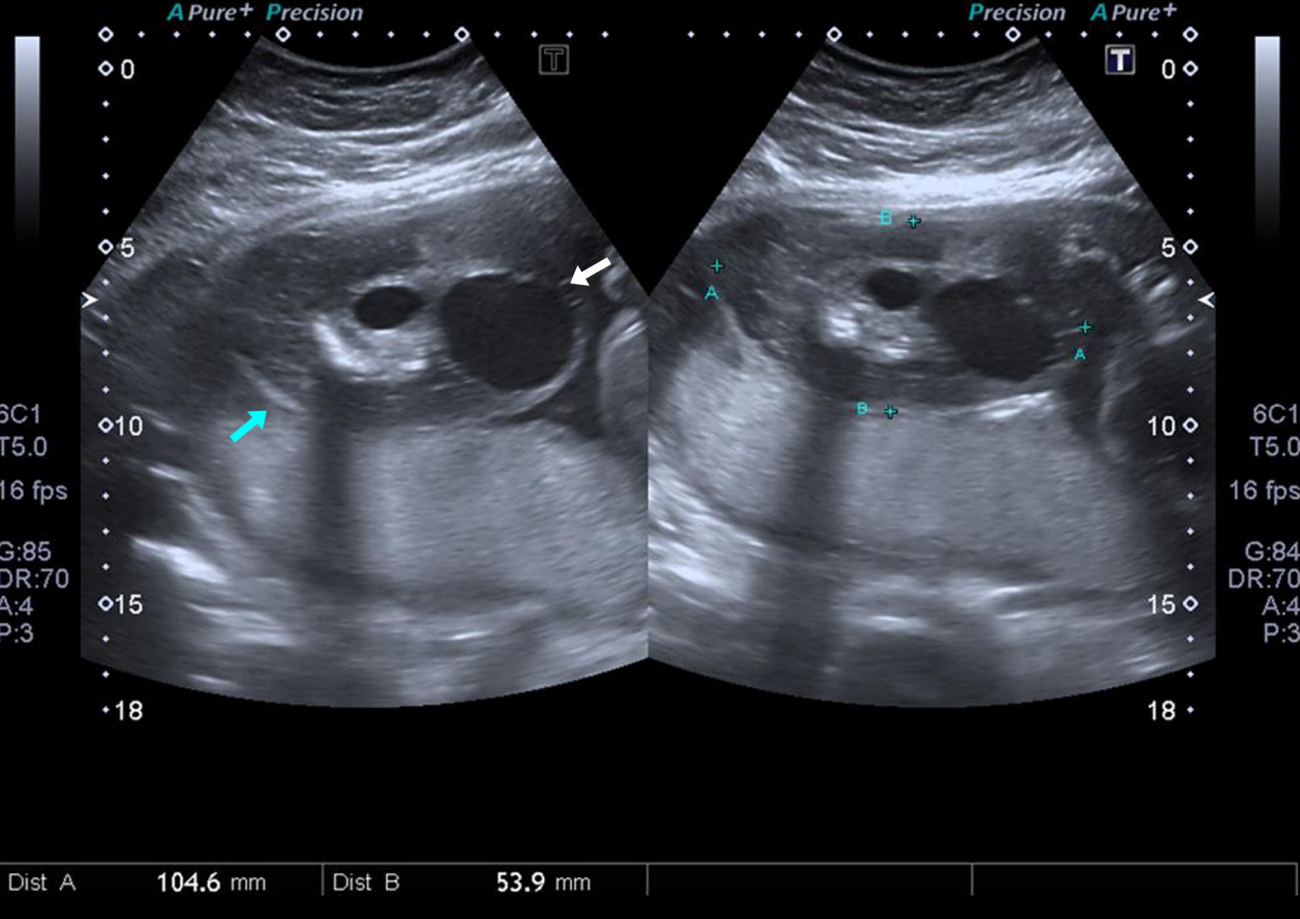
Second-trimester ultrasound scan. A complex multiloculated cystic mass with a larger cystic component (white arrow) and septations and calcifications (blue arrows) can be seen.
